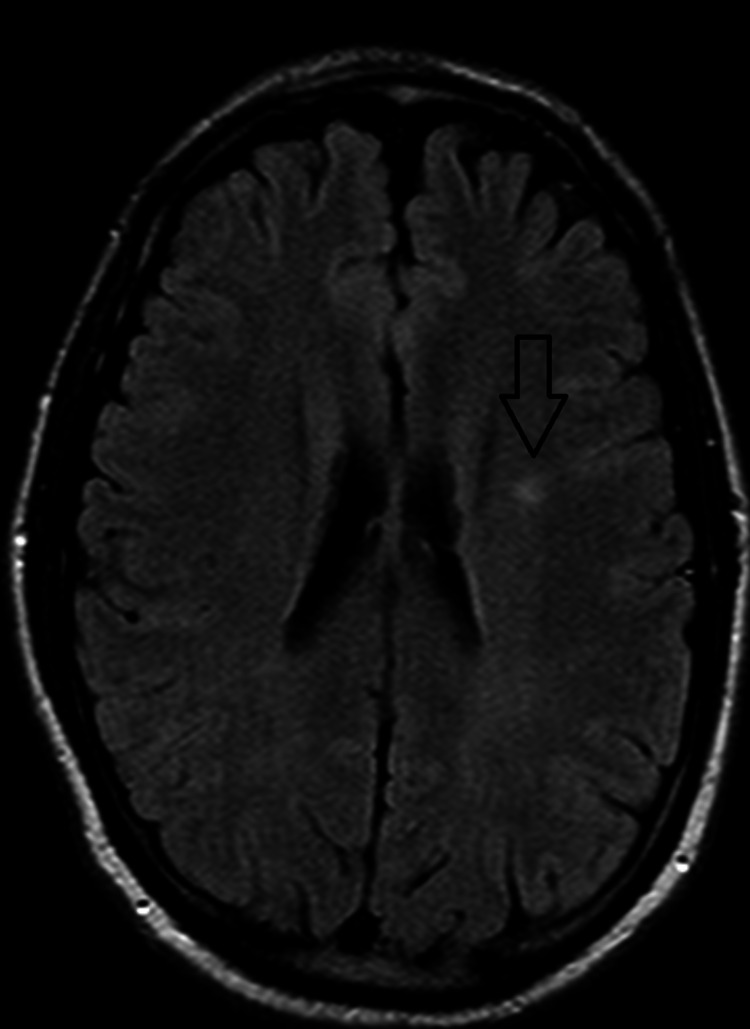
MRI of the head shows a small nonspecific focus of increased signal intensity in the left posterior frontal periventricular white matter, possibly representing a small focus of demyelination, chronic ischemia, or gliosis
